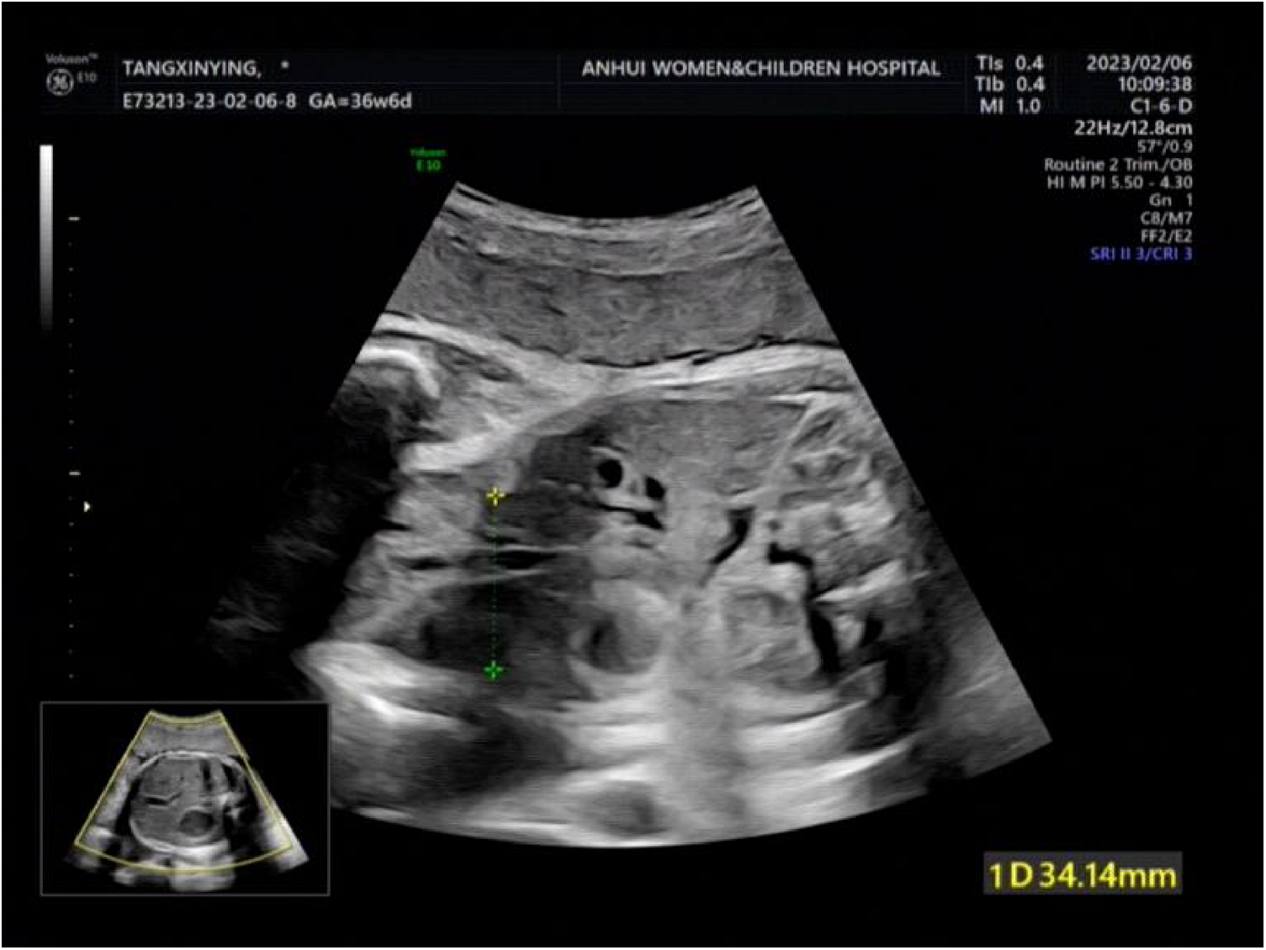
The targeted quaternary ultrasound of our hospital showed that 34 mm abdominal wall defect or abdominal wall continuity was interrupted.
